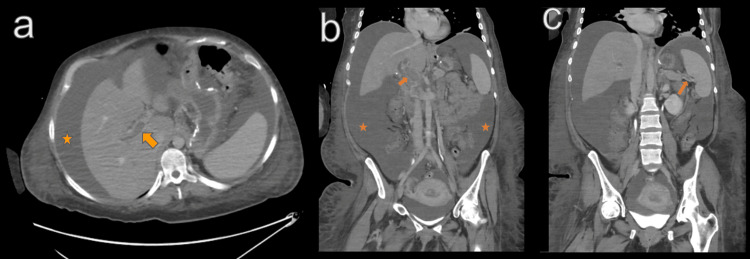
Axial (a) and coronal (b, c) contrast-enhanced CT of the abdomen demonstrating extensive portal vein and splenic vein thrombosis (arrows) and extensive abdominal ascites (star).
